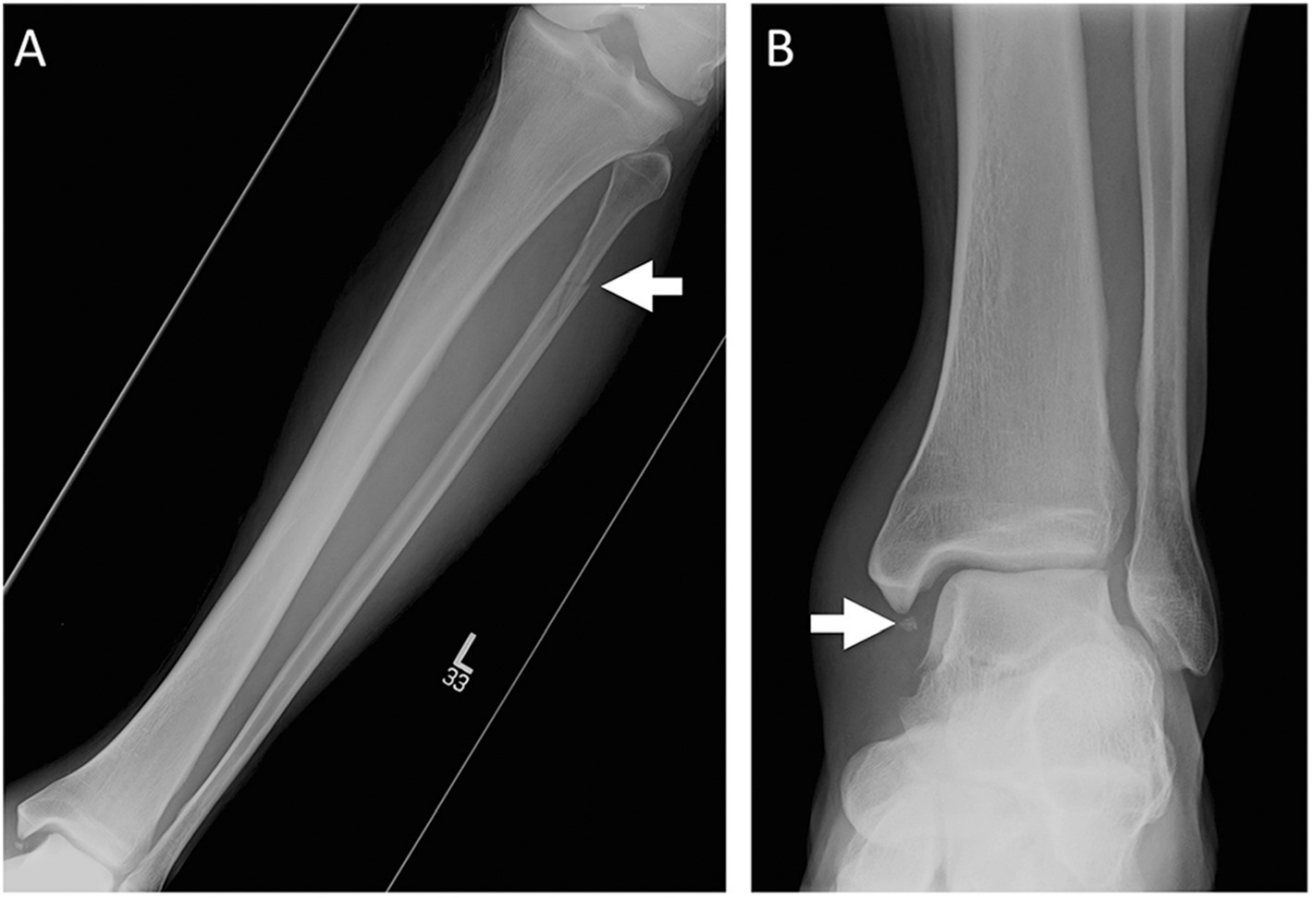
Maisonneuve fracture. a AP tibula/fibula radiograph with minimally displaced fracture of the proximal fibular shaft (*arrow*). b AP ankle radiograph in the same patient. Soft tissue swelling overlying the lateral malleolus, with avulsion fracture of the inferior aspect of the medial malleolus (*arrow*). This patient had confirmed disruption of the syndesmosis, with subsequent syndesmotic fixation
